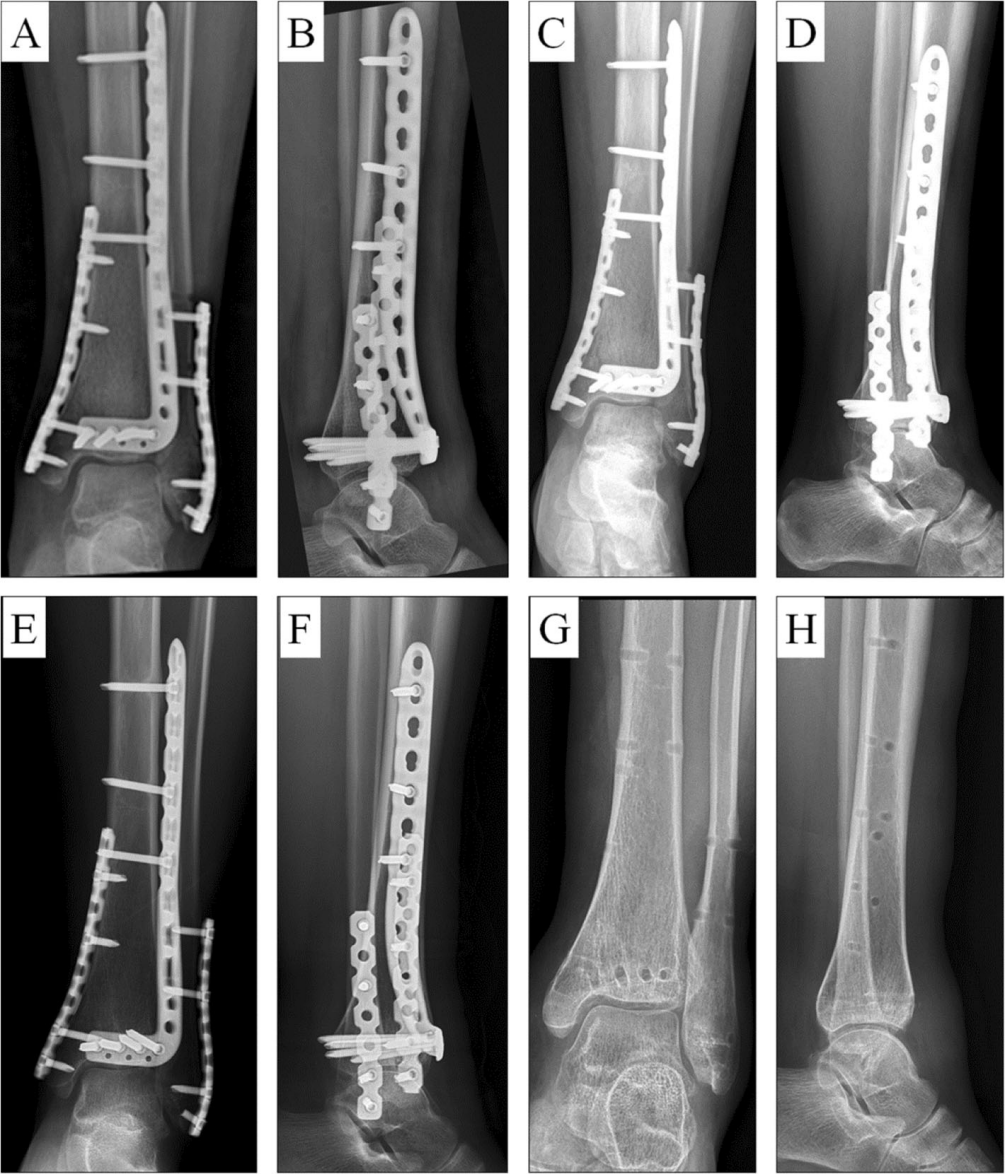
Postoperative AP and lateral X-ray examinations of the patient at a, b 1 month, c, d 3 months, and e, f 6 months postoperatively, as well as g, h after implant removal
